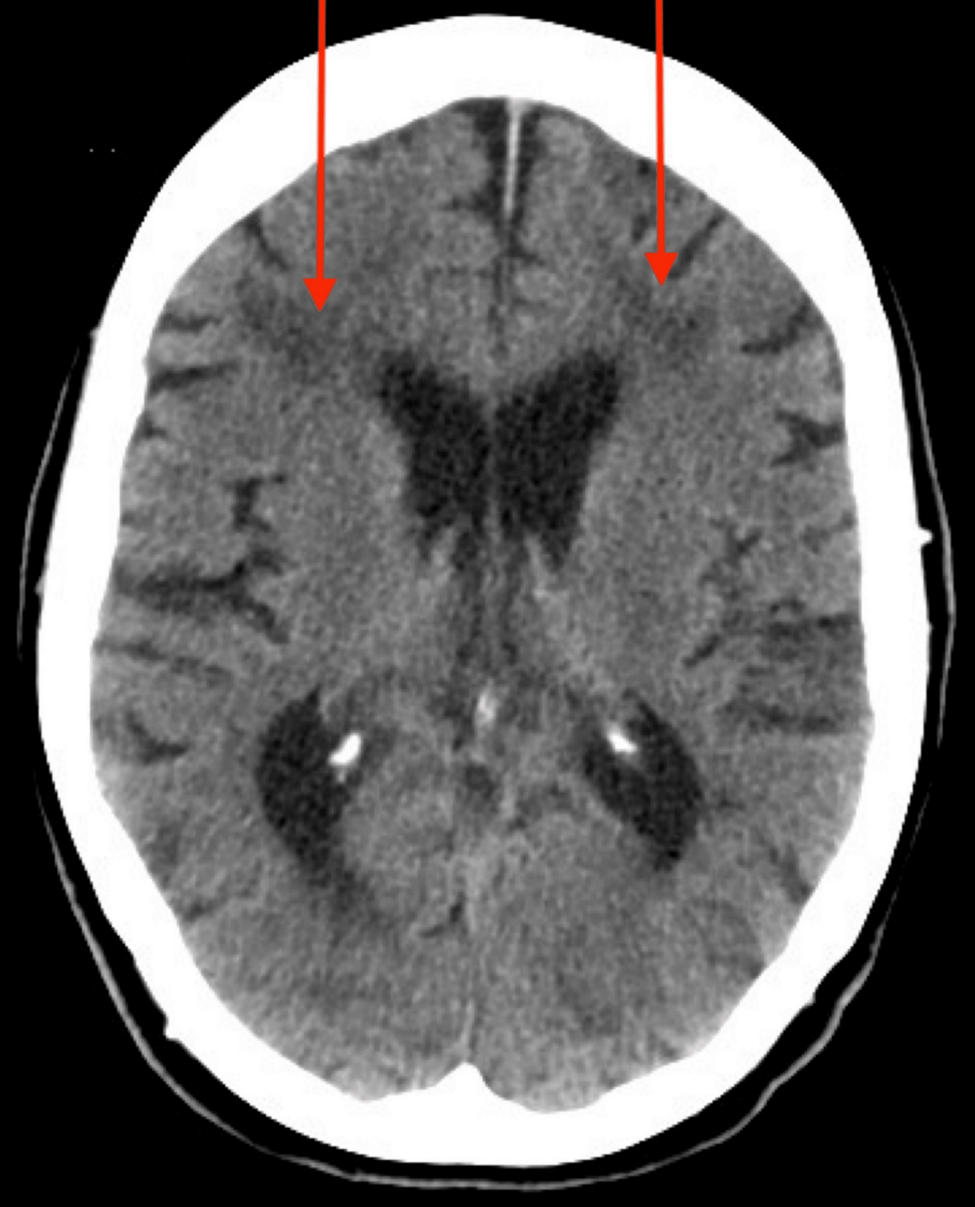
Microvascular ischemic changes Axial CT scan of the brain revealing mild, nonspecific low-attenuation white matter changes bilaterally (red arrows), likely representing chronic small vessel ischemic disease.
